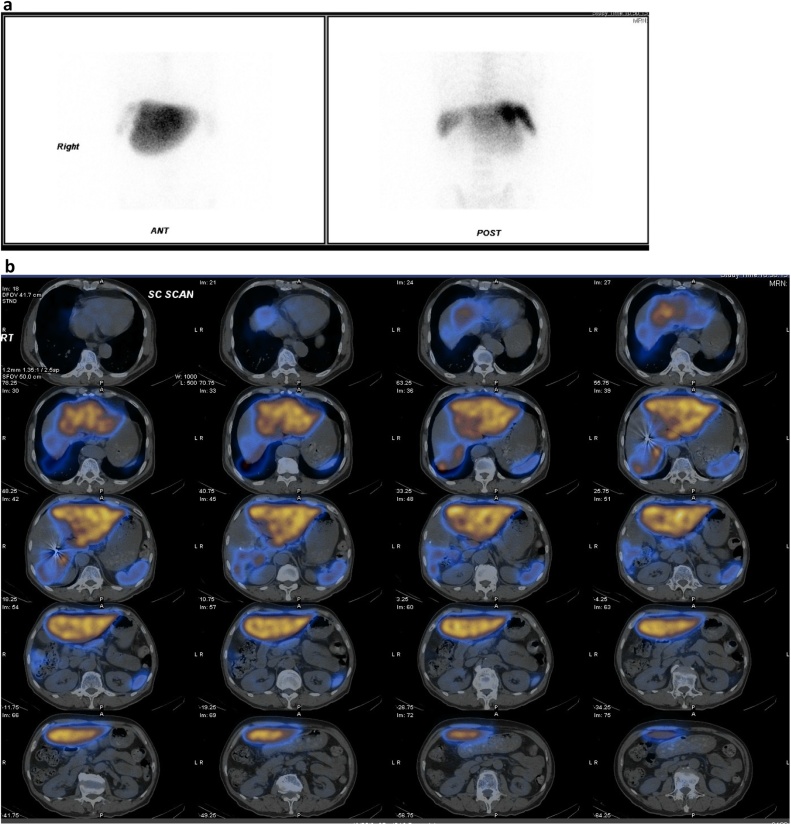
a) Planar view of abdomen in Tc-99 m colloid scintigraphy. Most of the colloid localized in the liver and spleen; only minimal uptake was found on the right lobe and a small amount on bone marrow. b) Fusion image of SPECT-CT in Tc-99 m sulfur colloid scintigraphy. The hypertrophic left lobe took up most of Tc-99 m SC injected; only minimal uptake was found on the right lobe.
